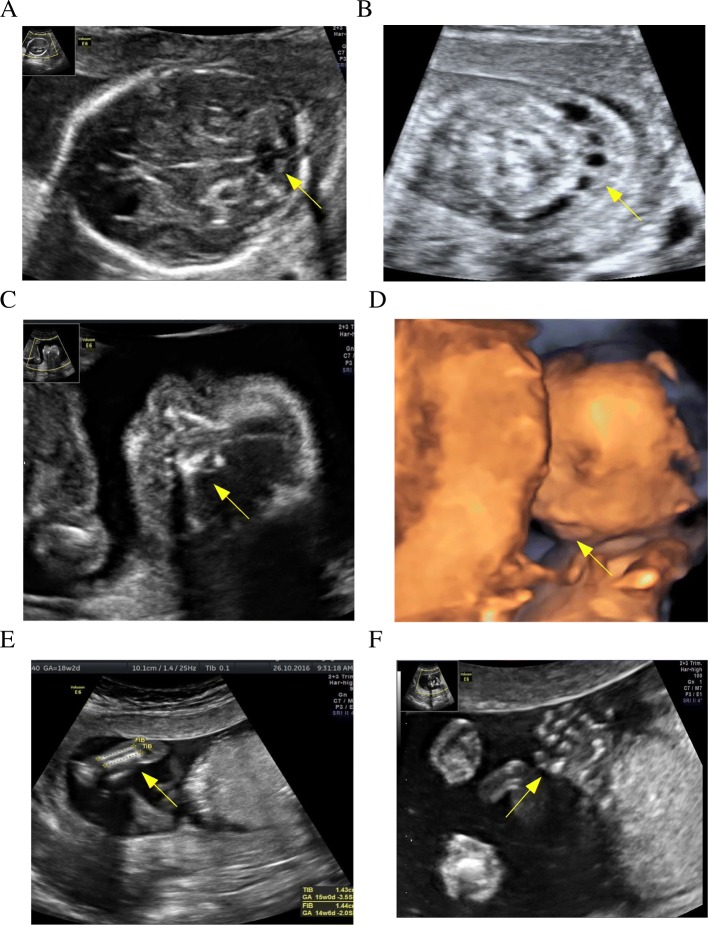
Abnormal foetal image in ultrasound examination at 18 weeks of gestation. a - hypoplasia of the cerebellar vermis, absent cavum septum pellucidum, b - cystic hygroma, c - hypertelorism, abnormal nostrils, broad nasal root, d - retrognathia, e - shortening of the long bones, f - clinodactyly of the 5th finger
